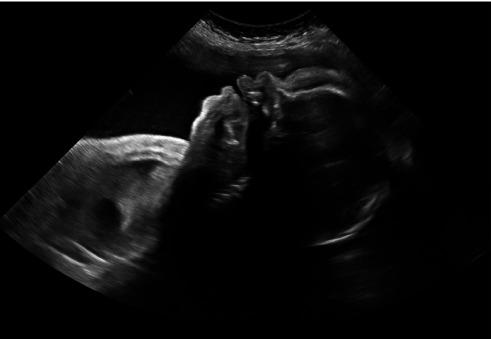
Ultrasound demonstrating worsening scalp and neck edema.
